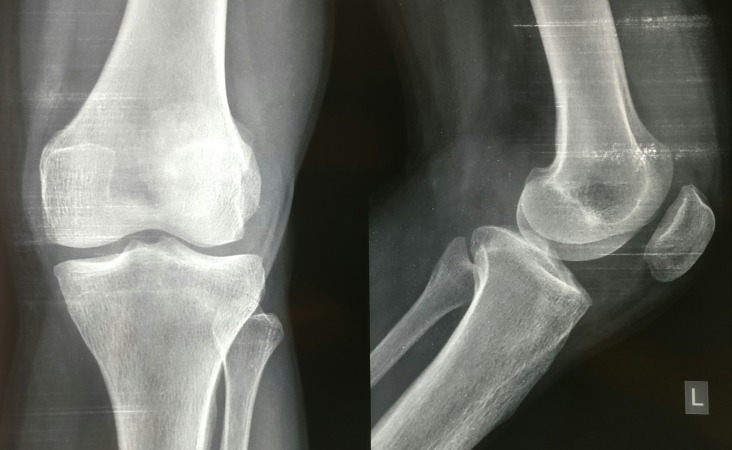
Pre-op radiograph of knee joint showing no abnormality
